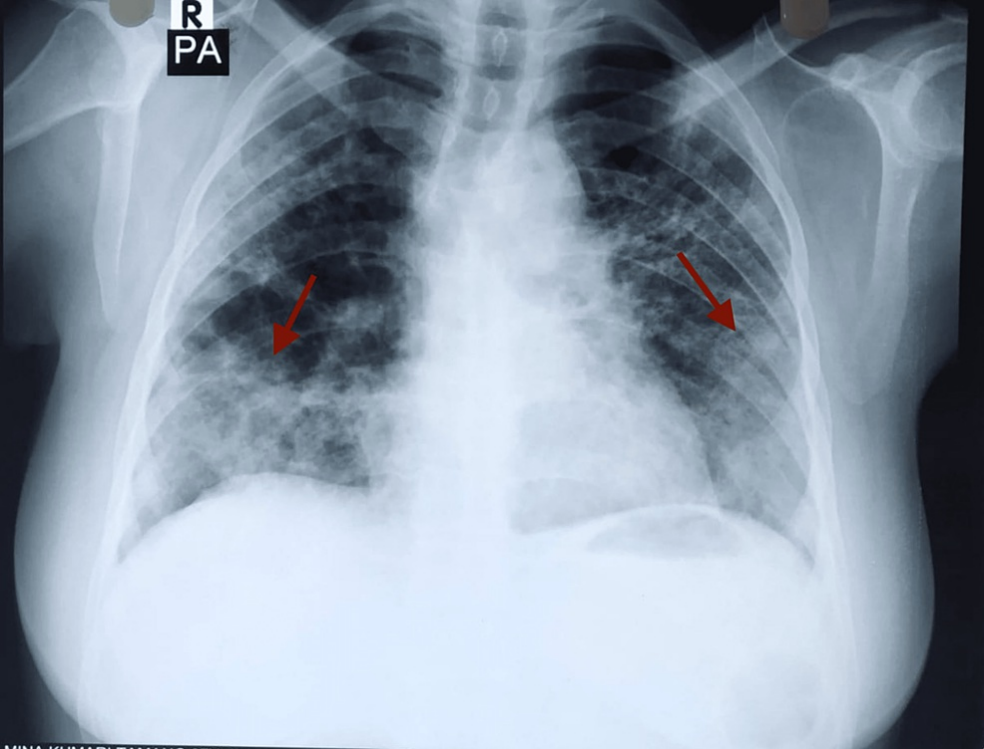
Chest x-ray showing bilateral consolidation
